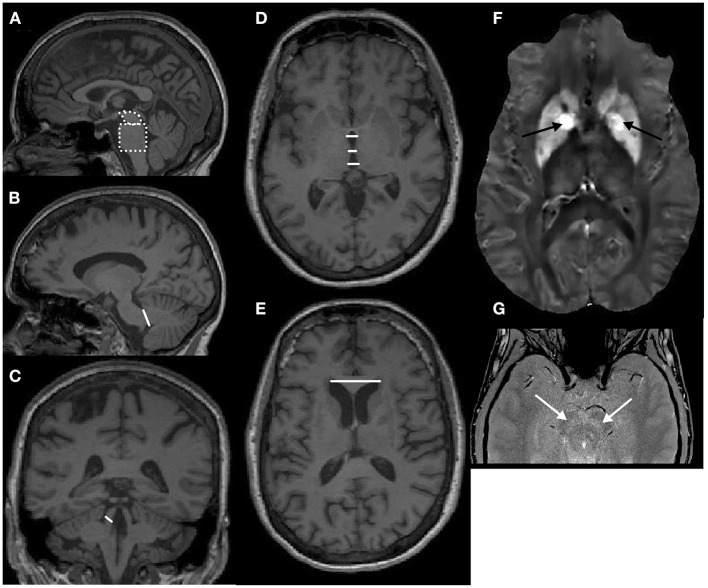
(A–E) T1-weighted anatomical images demonstrating the landmarks for the calculation of the original magnetic resonance parkinsonism index (MRPI) and MRPI 2.0 (see the formulae in the main text). (A) The midbrain and pons areas outlined with white dotted lines on a sagittal image. (B) Middle cerebellar peduncle width marked with a white line on a sagittal image. (C) Superior cerebellar peduncle width marked with a white line on a coronal image. (D) Third ventricle width (anterior, middle, and posterior) marked with white lines on an axial image at the anterior commissure—posterior commissure level. (E) Maximum left-to-right frontal horn width marked with a white line on an axial image. (F) Quantitative susceptibility mapping: black arrows point to the iron depositions (bright areas) in the globus pallidus bilaterally on an axial image. (G) Neuromelanin scan using magnetization transfer gradient echo sequences: white arrows point to the bilateral neuromelanin-rich substantia nigra (hyperintense) on an axial image.
